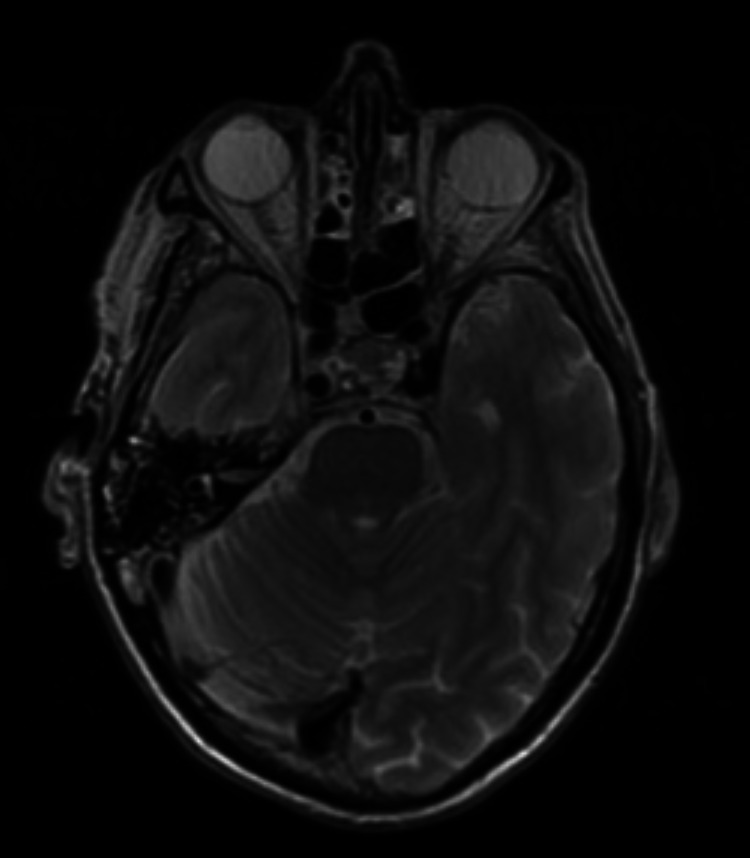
Repeat MRI brain and neck, three months after initial scan – T2-weighted.
